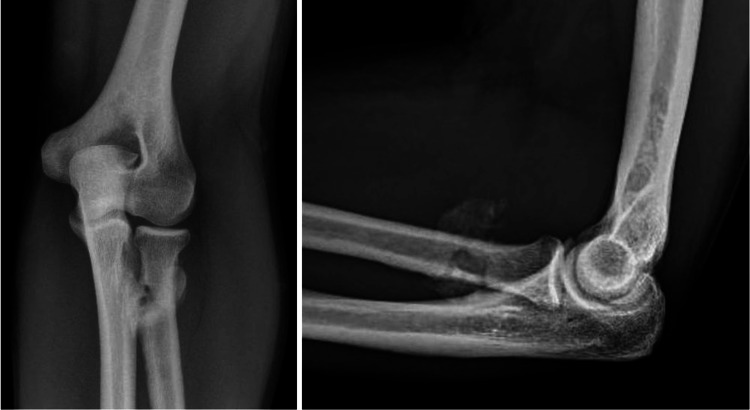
Anteroposterior and posterior X-ray view (author's original image) Heterotopic ossification identified six weeks after distal biceps tendon repair.
